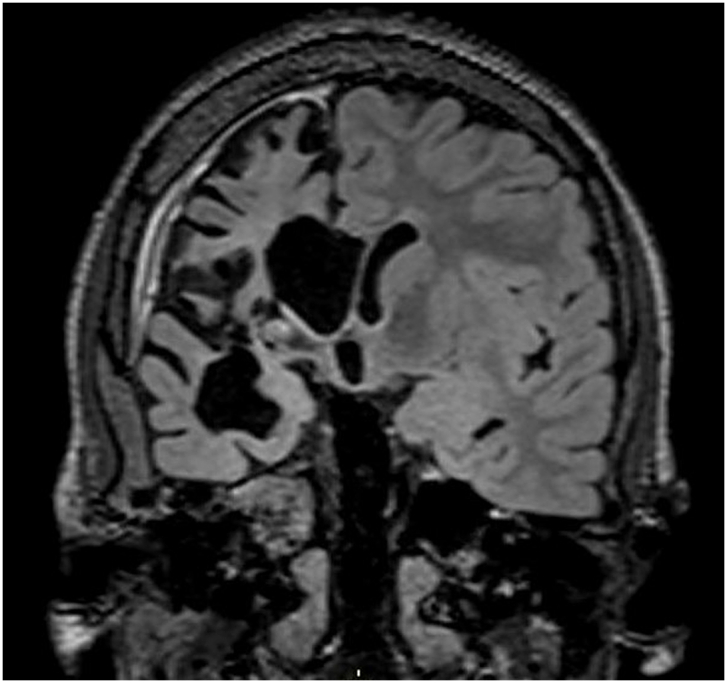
Patient 2 modified coronal sections using FLAIR sequences showing possible persisting connection exiting the right amygdala on pre-amygdalectomy MRI.
